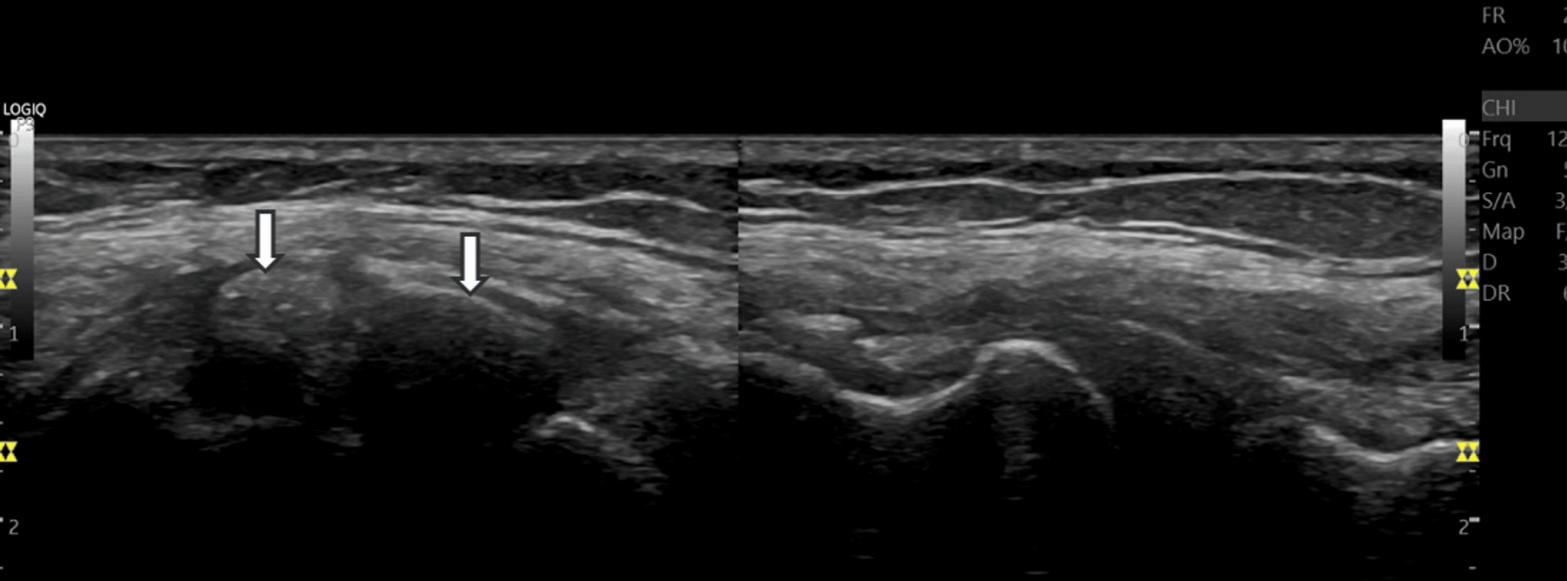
Comparative ultrasound image Comparative assessment with asymptomatic left knee (right half of the image), where LCL demonstrates a normal fibrillar and echoic pattern, with intact fibers and no evidence of calcification or thickening LCL: lateral collateral ligament
